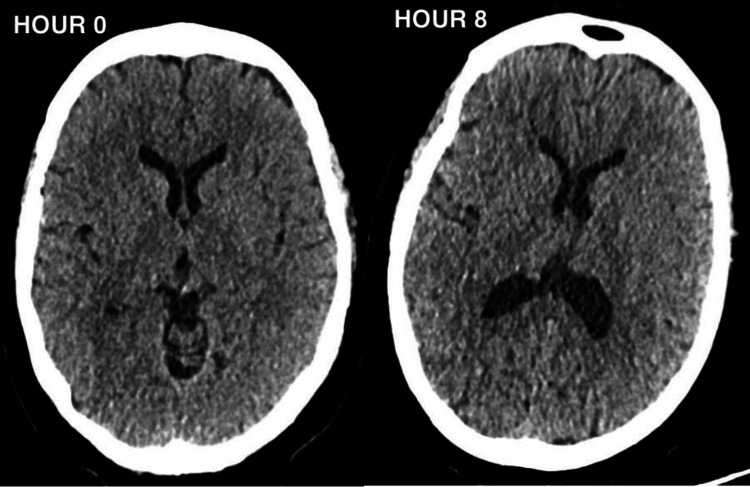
Showing the CT head at presentation (Hour 0) and eight hours later (Hour 8).
